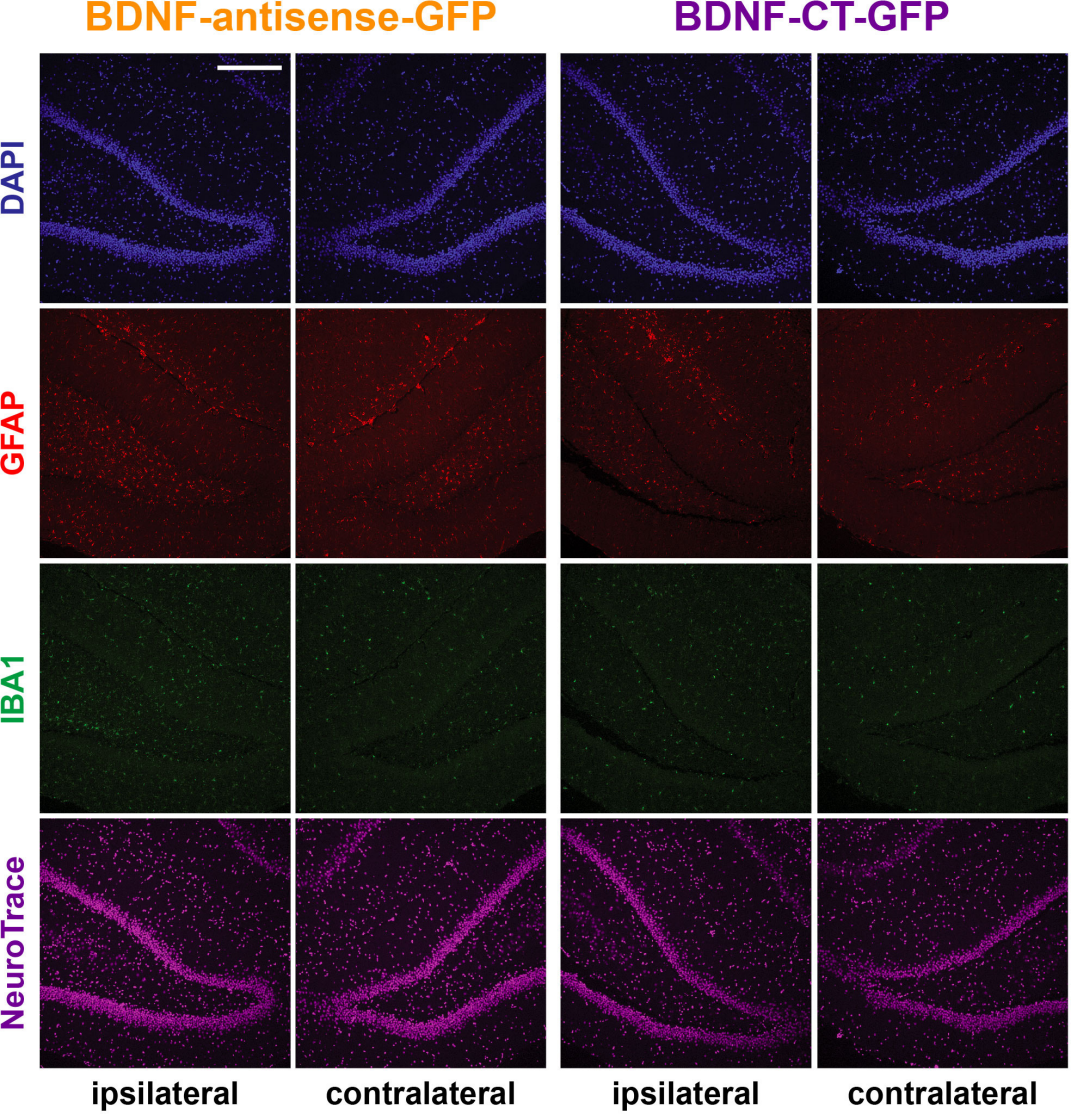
Absence of overt amplicon vector-induced toxicity after injection in the dorsal hippocampus. Dentate gyrus (DG) of the dorsal hippocampus injected (ipsilateral) and non-injected (controlateral) with BDNF-antisense-GFP or with BDNF-CT-GFP amplicon vector. Nuclei are marked by DAPI in blue, GFAP-positive astrocytes in red, IBA-1-positive microglia in green and neuronal nuclei are labeled by NeuroTrace in magenta. Horizontal bars = 100 μm.
